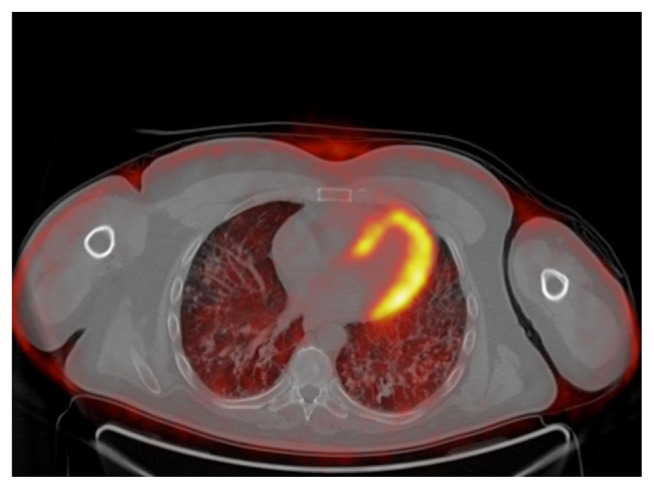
PET/CT examination: not significant increase in metabolic activity in both lungs.
